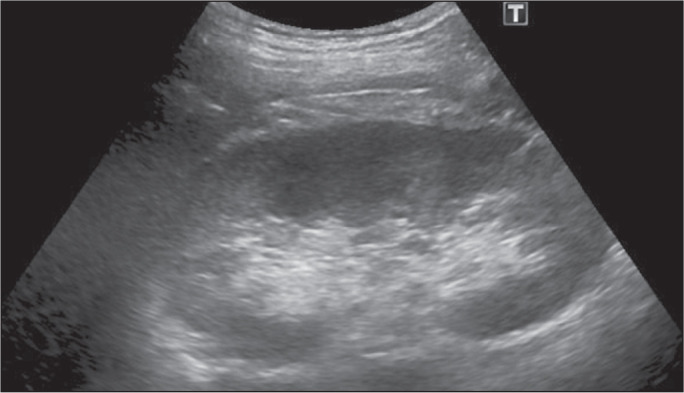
Ultrasound examination at 6 months of follow-up, showing complete
